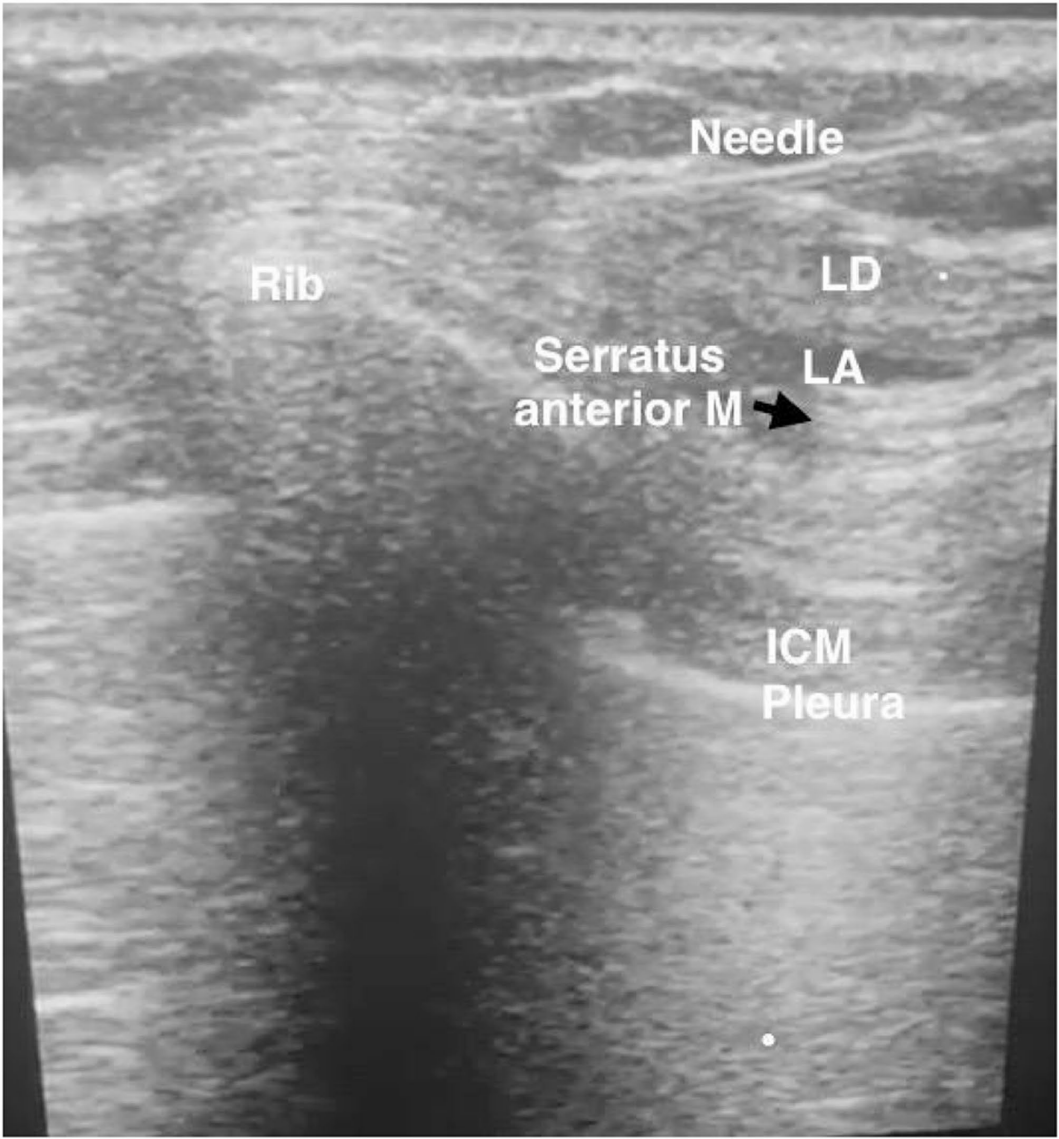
Sonographic image of the superficial serratus anterior plane block. LD: latissimus dorsi muscle, ICM: intercostal muscle, LA: local anesthetic spread between the serratus, and LD muscles
